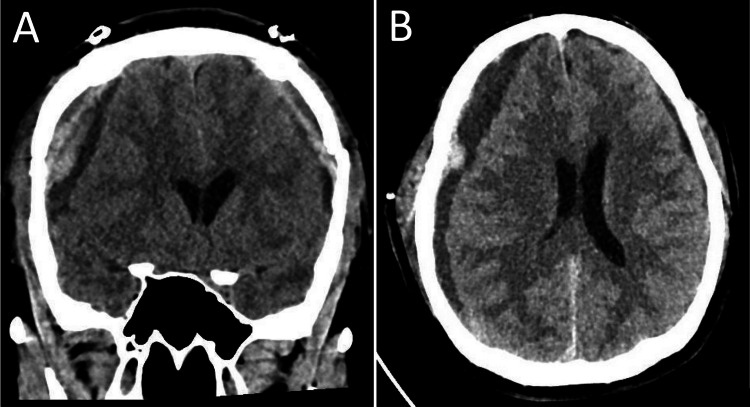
Plain cranial CT scan in (A) coronal and (B) axial views showing recurrence of subdural hematoma with leftward midline shift.
